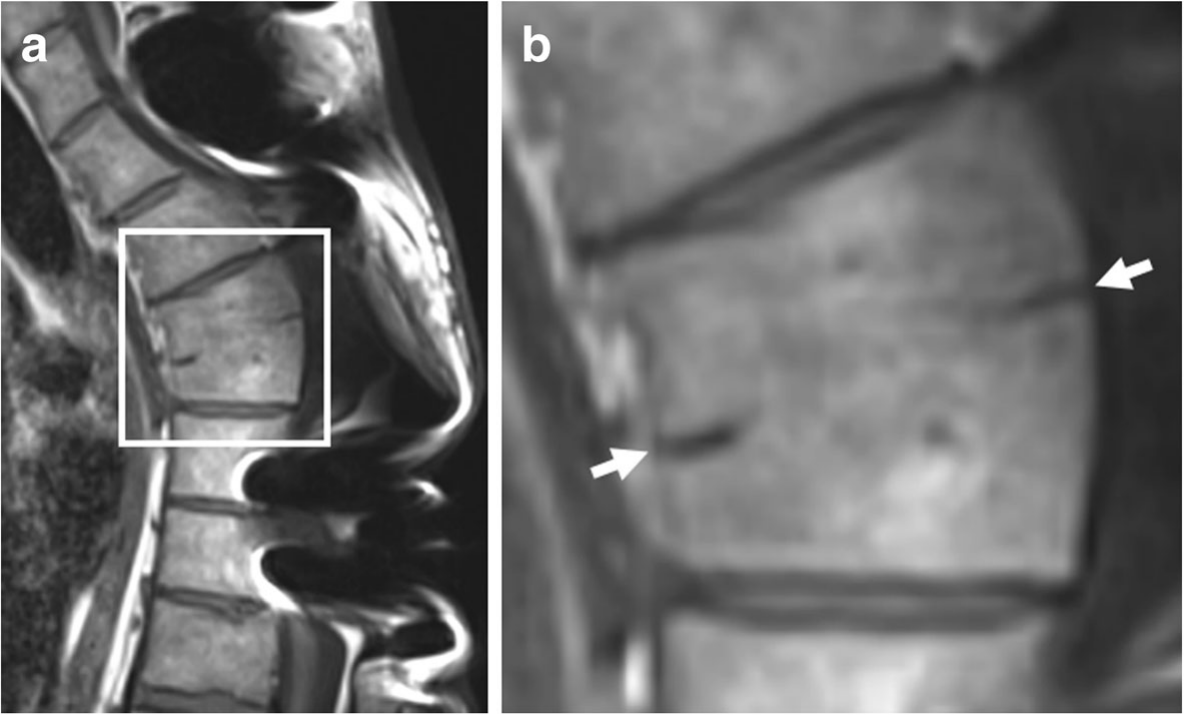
Pitfalls in imaging and diagnosis of new bone formation: post-traumatic vertebral fusion. Sagittal T1-weighted MR images show interbody vertebral fusion years after severe spinal trauma, with discrete remnants of the intervertebral disc (arrows). This image suggests that the original injury included both vertebral endplates and the intervertebral disc. Also note that the height is less than the sum of the two vertebral bodies and the intervertebral disc, and antero-posterior diameter is not narrowed, two features which help differentiate this image from a congenital block vertebra. Note: b is an enlargement of the region outlined in a
